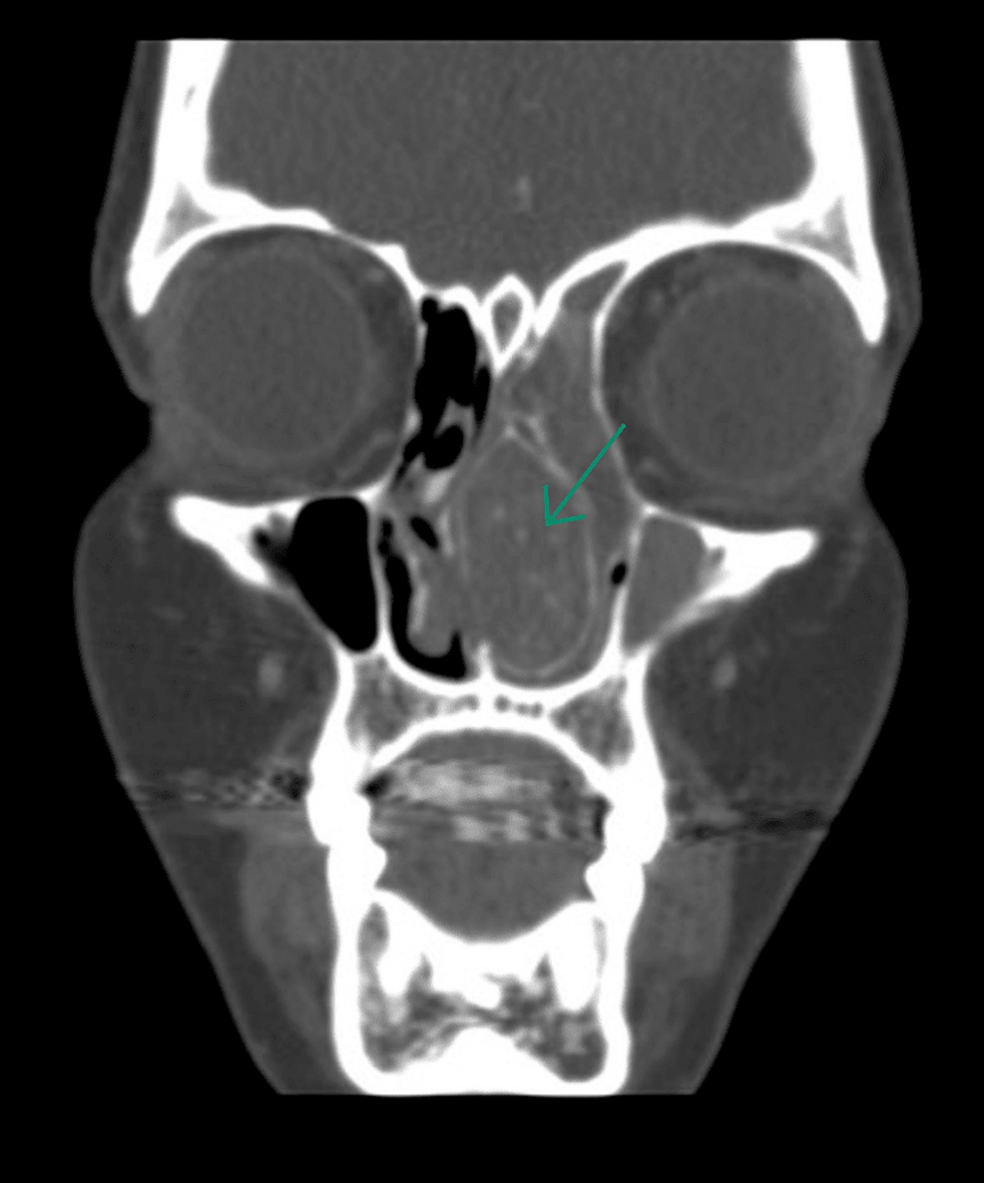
Coronal section of bone-window CT showing an expansile mass of 1.7 × 3.7 × 3.0 cm with central calcification and egg-shell border. The mass is obstructing the left nasal airway and causing compression over the septum.
